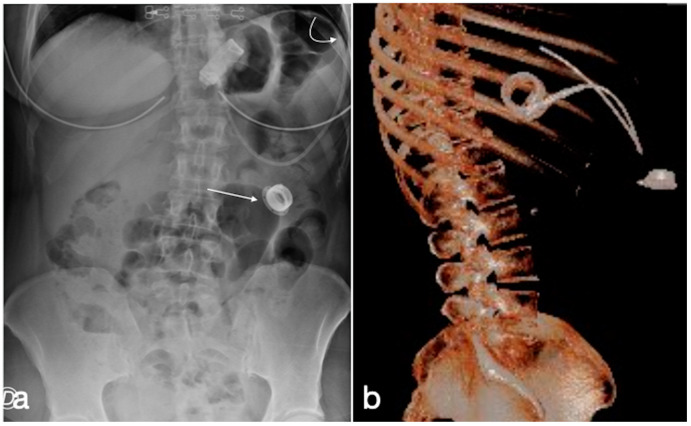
Gastric banding, detachment of the catheter from the port. A female patient in whom it was no longer possible to adjust the gastric banding. In the CT scout, the detachment of the catheter extremity (a, curved arrow) from the port (a, straight arrow) is clearly evident. In (b), the same findings are shown in the volume three-dimensional reconstruction.
